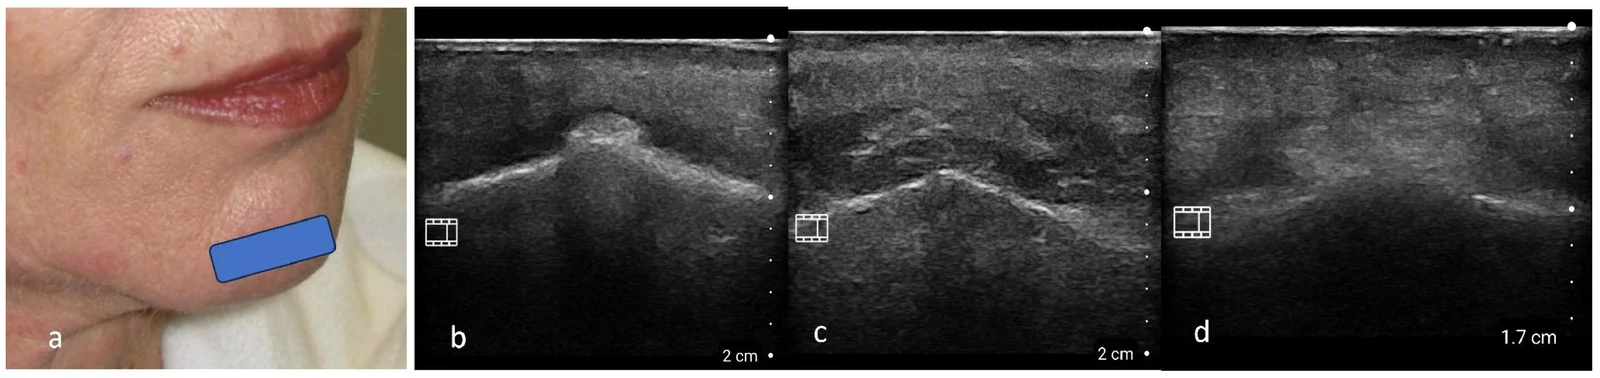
Ultrasonographic images of mentalis muscle: (a) The blue rectangle represents the position of the 20 MHz probe positioned horizontally on the chin. Three different types of images may be identified. (b) Type A-1 appears as a dome-shaped hypoechoic area that merges at a more superficial part of the chin. Between the two mentalis muscles, the hyperechoic connective-adipose tissue is well evident. (c) Type A-2 is dome-shaped, but the muscle fibres on each side are separate. (d) Type B: two separated hypoechoic areas are located lateral to the midline and are completely separated by connective fatty tissue.
